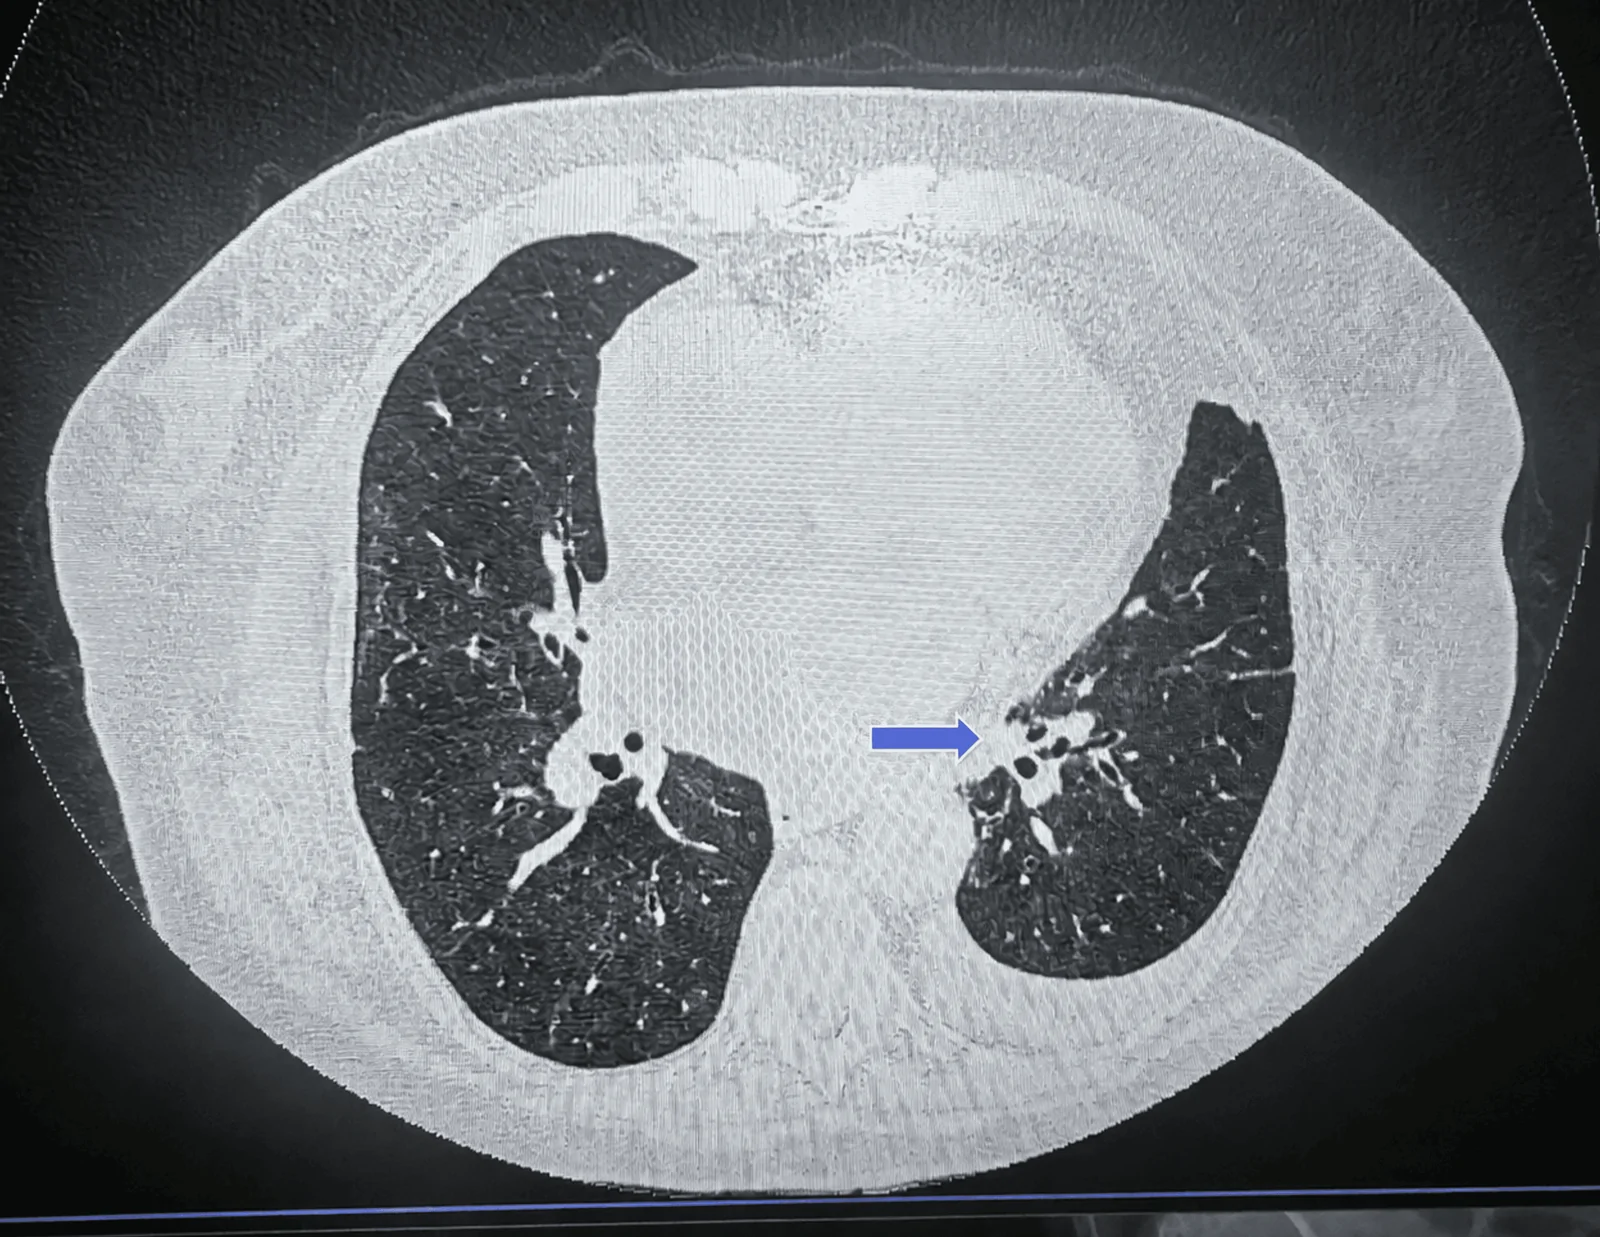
Axial chest CT image demonstrating left hilar lymphadenopathy The blue arrow indicates the enlarged left hilar lymph nodes. CT: computed tomography
